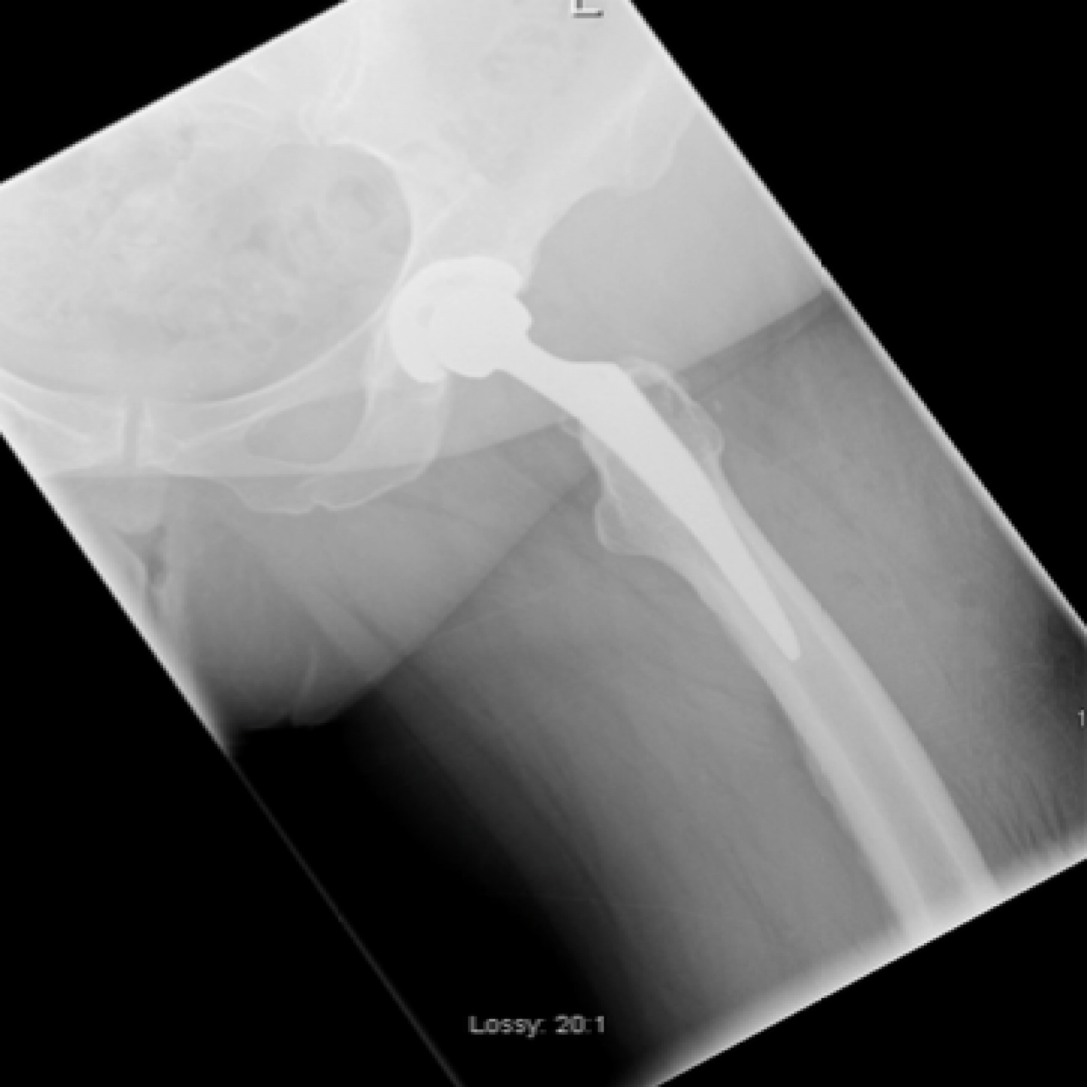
Post-operative lateral radiograph of her left hip post head and liner exchange with concentric placement of the femoral head in the acetabular cup
